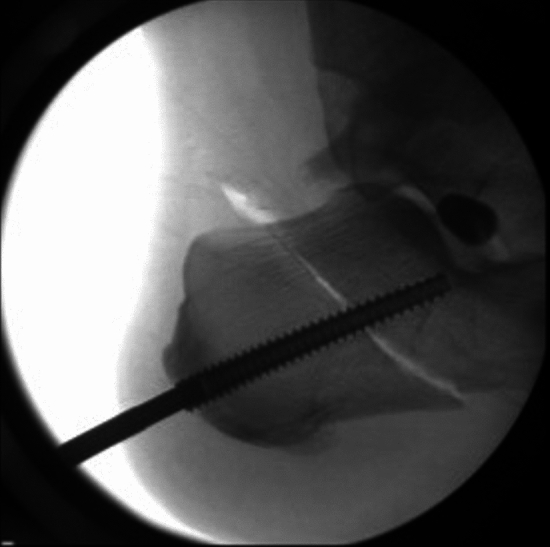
Lateral intra-operative radiograph demonstrating sinus tarsi implant position
